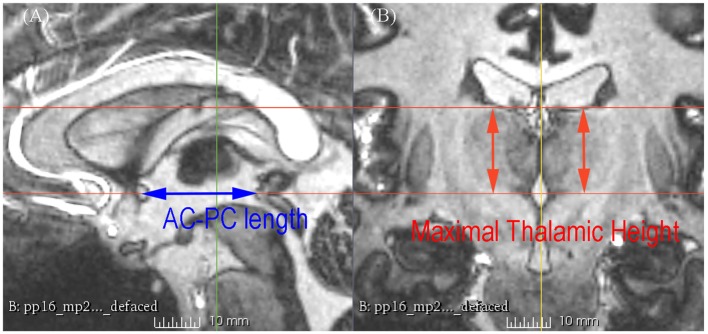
7 T MRI showing the measurement of the AC-posterior commissure (PC) length and the maximal thalamic height on the sagittal section (A) and the coronal section (B).
